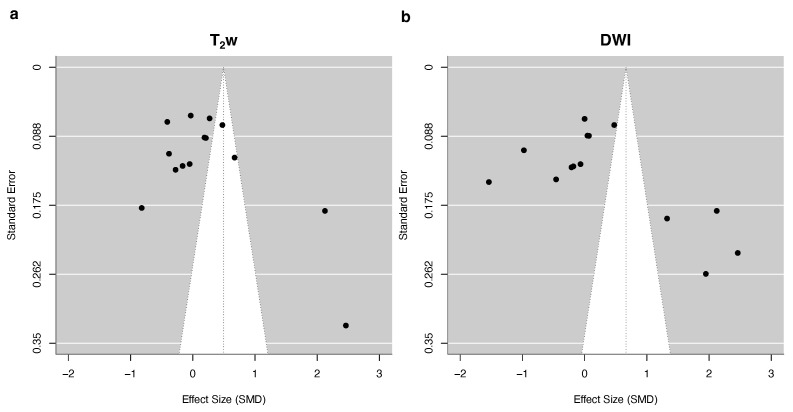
Funnel plots of the studies included in the meta-analysis of subjective image quality in T_2_w (a) and DWI (b).
